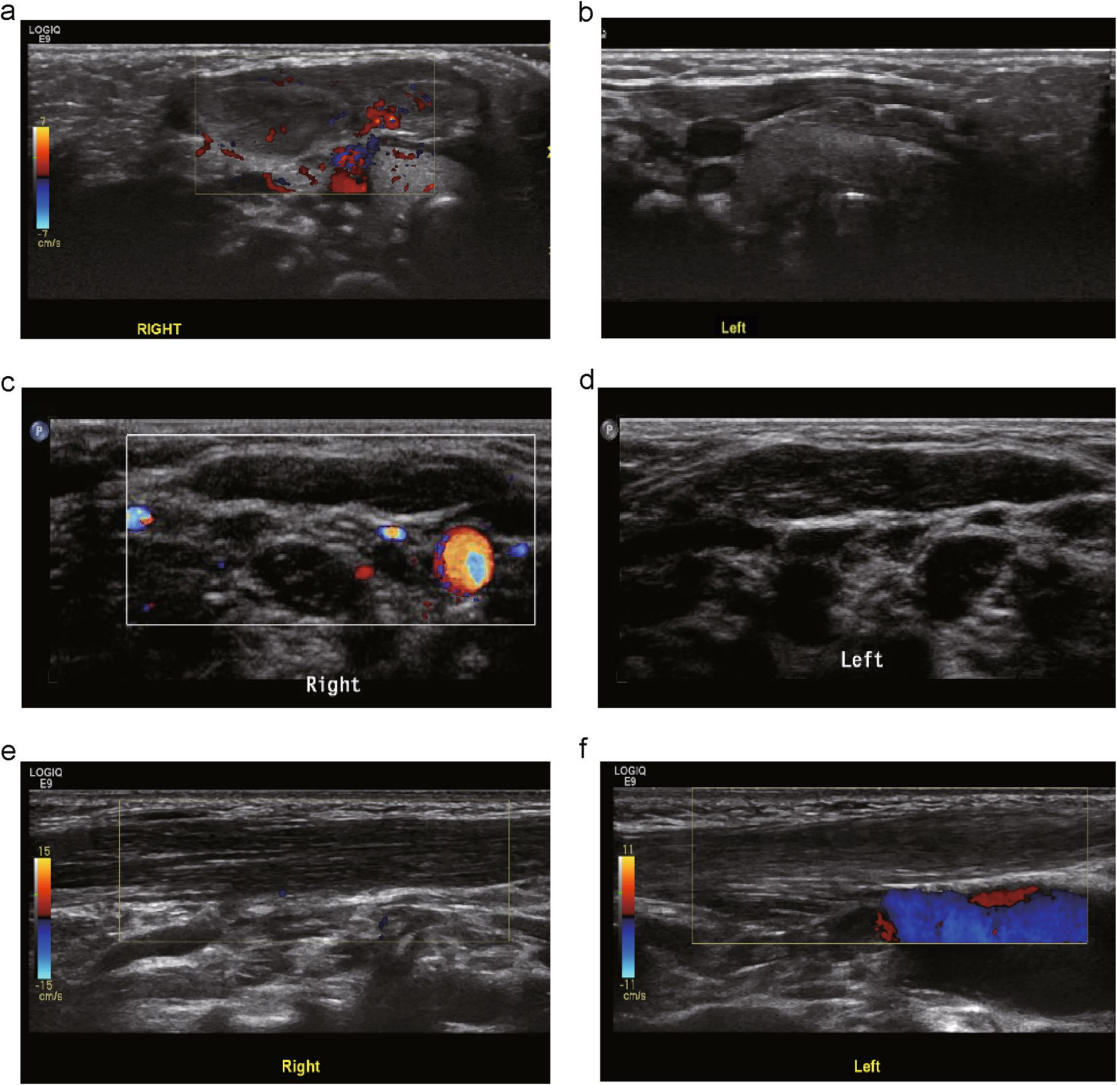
Imaging pictures of three types of ultrasound classification of CMT. a Local mass-type Ultrasound pictures of the affected side: the SCM of the affected side is thickened and nodular, with a size of about 3.4 × 3.0 × 1.1 cm. The echo is unevenly enhanced, the muscle texture is disordered, and the echo is unclear. b Local mass type Ultrasound pictures of the healthy side: the SCM echo of the healthy side shows no obvious abnormality, the muscle fiber texture is clear, and the thickness of the upper, middle and lower segments are 2.9 mm, 3.0 mm, and 3.0 mm, respectively. c Uniformly thickening type Ultrasound picture of the healthy side: no abnormality in the echo of the right SCM. The thickness of the middle section is 3.2 mm. d Uniformly thickening type Ultrasound picture of the affected side: the echo of the left SCM is enhanced and thickened, mainly in the lower part of the sternal bundle, and the thickness of the middle part is 4.0 mm. e Ultrasound image of the healthy side of atrophy type: the SCM echo of the healthy side (right) shows no abnormality, the muscle fiber texture is clear, and the thickness of the middle section is 7.3 mm. f Ultrasound image of the affected side of atrophy type: the affected side (left) has enhanced SCM echoes, mainly the sternal bundle, with a thickness of 5.2 mm in the middle
